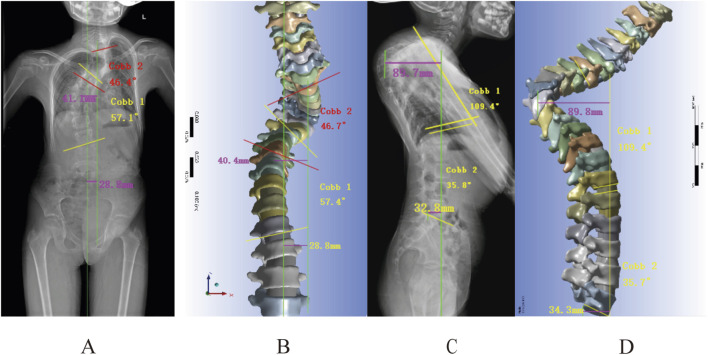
Geometric measurements versus radiographic findings: (A,B) Frontal radiograph measurements: Cobb angle, apical vertebral translation, and coronal balance parameters; (C,D) Lateral radiograph measurements of kyphotic Cobb, lumbar lordosis, and sagittal vertical axis (SVA).
